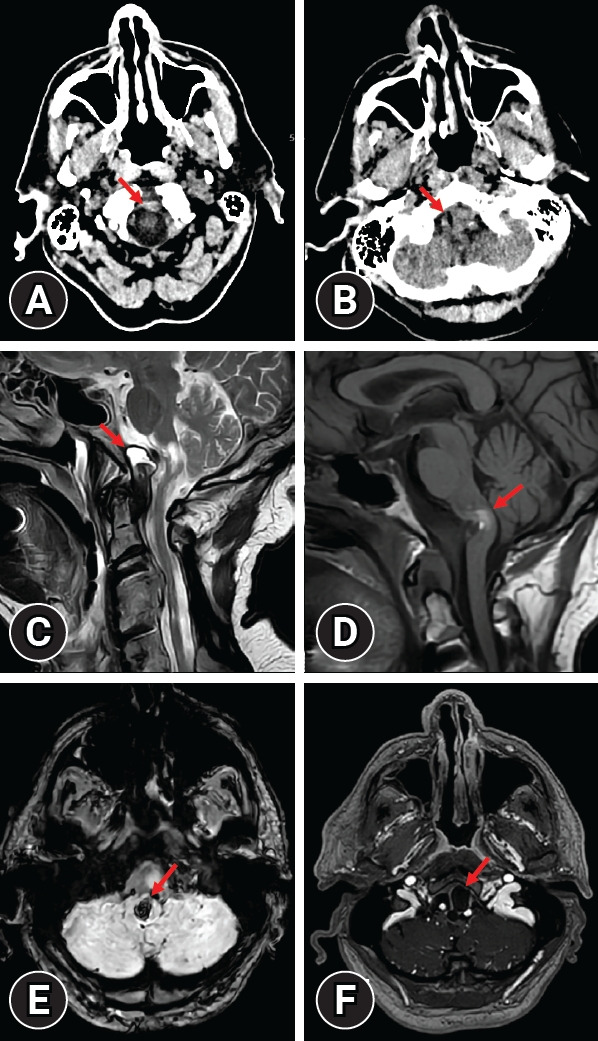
(A, B) Axial non-contrast computed tomography images demonstrate a well-defined extra-axial cystic lesion at the craniocervical junction, centered between the clivus and the odontoid process (arrows), causing posterior displacement and marked ventral compression of the medulla oblongata, without evidence of acute intracranial hemorrhage. (C) Sagittal T2-weighted magnetic resonance imaging (MRI) shows a heterogeneous hyperintense cystic lesion arising from the atlanto-odontoid joint (arrow), with superior extension into the pre-bulbar cistern. (D) Sagittal non-contrast T1-weighted MRI demonstrates mass effect with anterior indentation and deformation of the ventral aspect of the medulla (arrow), extending into the pre-pontine cistern. (E) Axial non-contrast T1-weighted MRI reveals intrinsic hyperintense signals within the medulla oblongata (arrow), consistent with subacute intra-axial hemorrhage, associated with surrounding vasogenic edema. (F) Axial post-contrast T1-weighted MRI with subtraction shows no internal solid enhancement in the lesion (arrow), supporting the cystic and non-neoplastic nature of the lesion.
